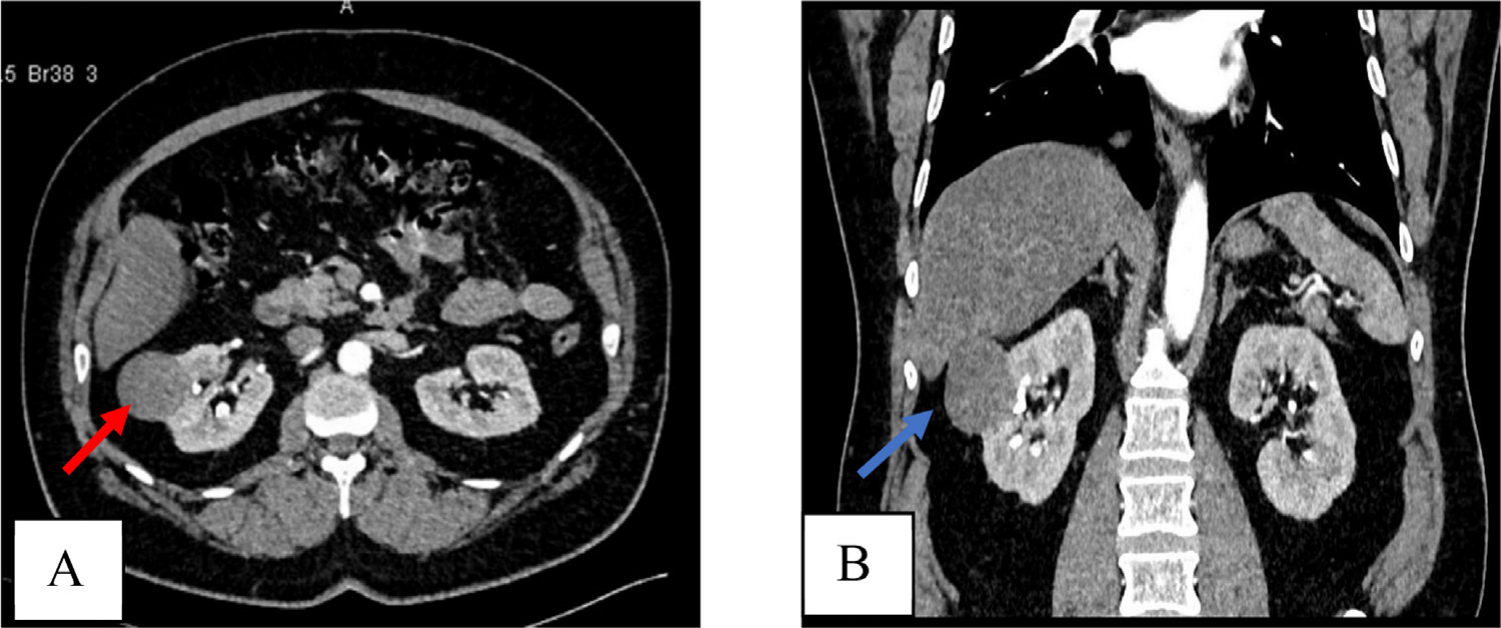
Contrasted CT scan showing a 5 cm mass in the right kidney on (A) cross sectional plane (red arrow) and (B) Coronal section (blue arrow).
